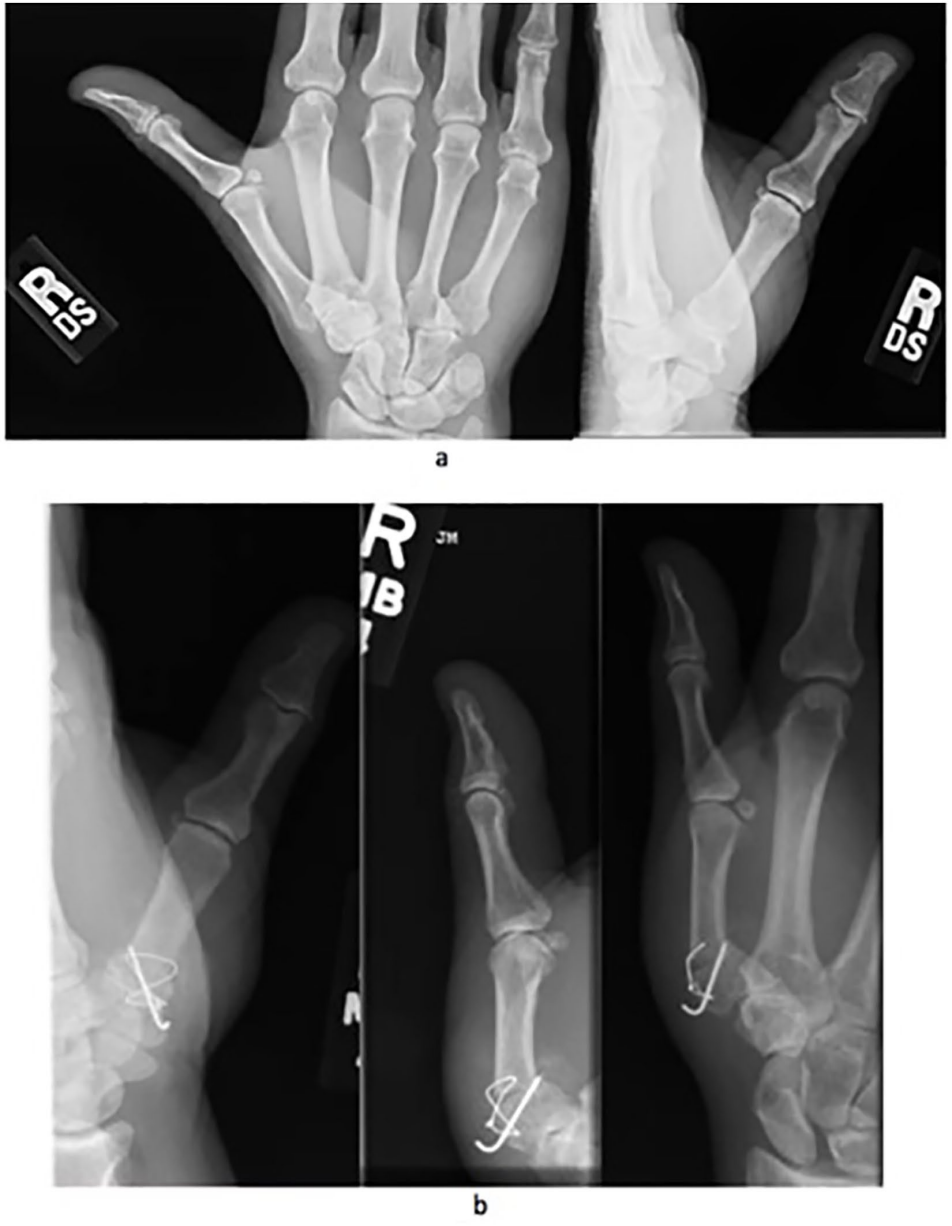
Radiographs of a patient with Wilson osteotomy: (a) preoperative and (b) postoperative.
